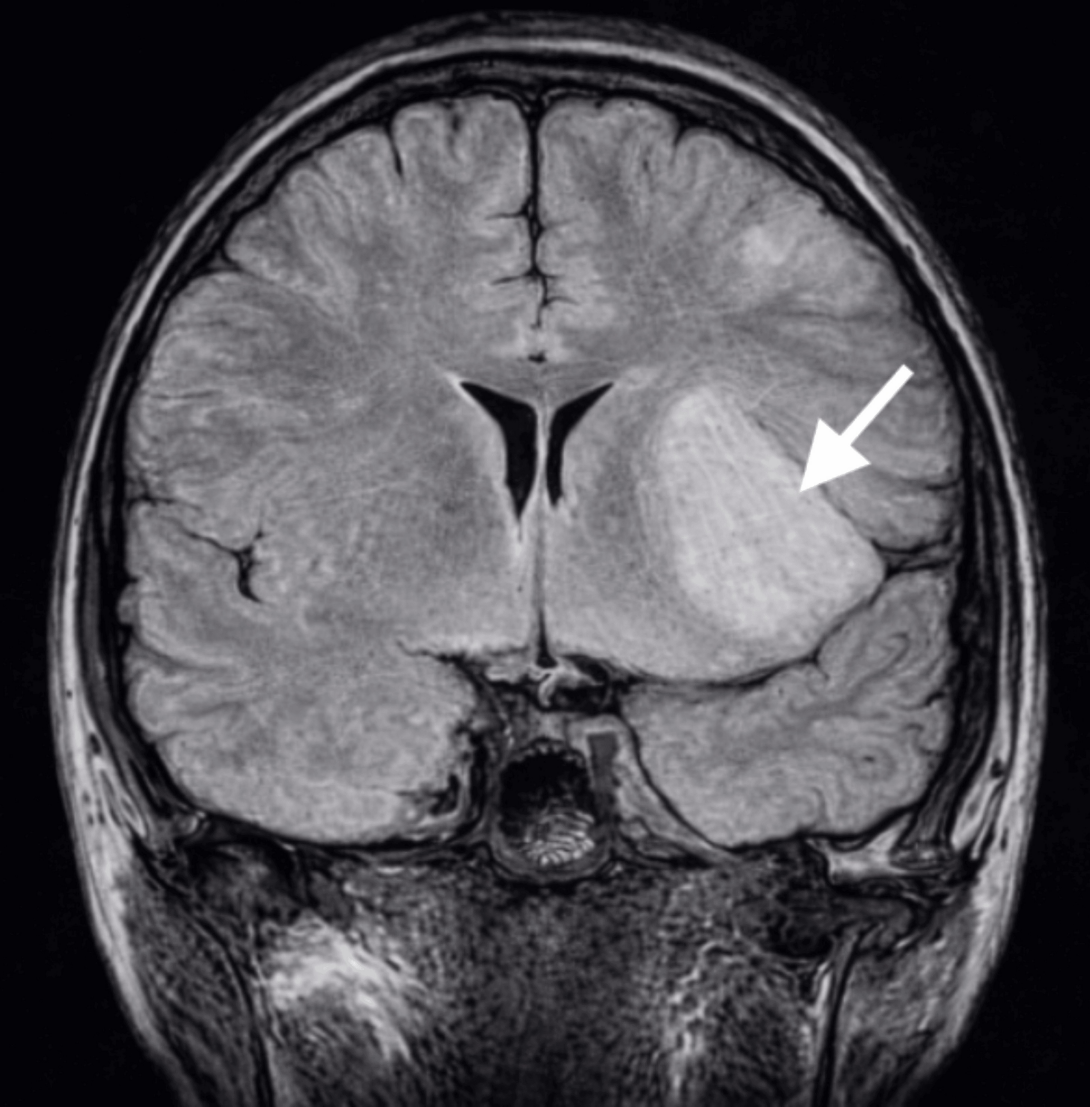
Coronal FLAIR MRI of the brain without contrast demonstrates a poorly circumscribed, FLAIR-hyperintense lesion in the left anterior aspect of the external capsule extending into the anterior insular cortex and frontal white matter (white arrow), imaging features suggestive of a low-grade astrocytoma FLAIR: fluid-attenuated inversion recovery; MRI: magnetic resonance imaging
